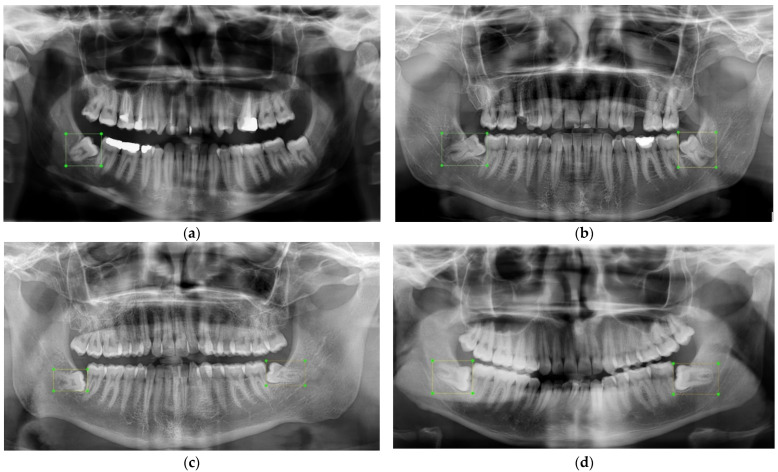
Examples of panoramic radiographs with bounding boxes. (a) One impacted tooth—me-sioangular left, (b) two impacted teeth—mesioangular left and right, (c,d) horizontal left and right.
